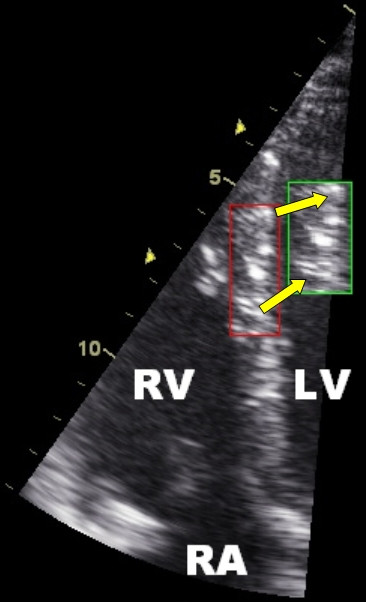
Typical speckled pattern of the myocardium on ultrasound in the septal wall. The tracking algorithm follows a unique speckle pattern during the cardiac cycle. The red square represents the starting location and the green square the location of the pattern at end systole. Note the change in distance between the speckles due to deformation (longitudinal shortening and radial thickening of the square). RV = right ventricle; LV = left ventricle; RA = right atrium.
